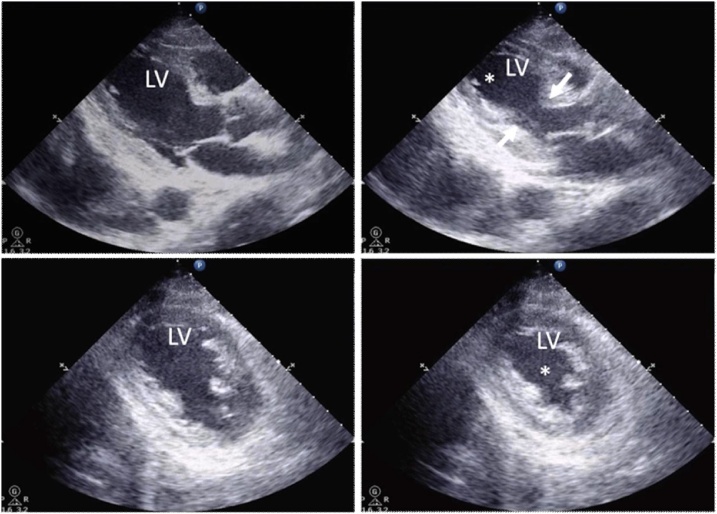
Echocardiogram on POD 4. Parasternal long axis views (top) and short axis views (bottom) of end-systolic frames (right) and end-diastolic frames (left) are shown. While the basal septum shows normokinesia (arrows), the apical septum is akinetic (*). LV; left ventricular.
